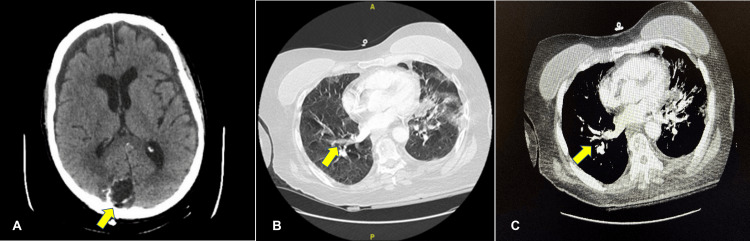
Post-treatment CT images of the patient's brain and chest A. CT scan of the brain post right occipital craniotomy. B. CT angiography of the chest with subsegmental pulmonary emboli in the right upper lobe. C. CT angiography of the chest axial view of the pulmonary emboli. CT, computed tomography
